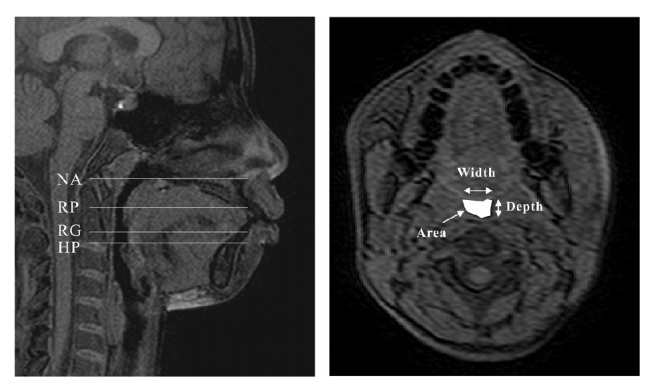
Landmarks and measurements of the upper airway on MRI. The nasopharynx (NA) was measured on the axial cross-section of the airway passing through the palatal plane. The retropalatal oropharynx (RP) was measured on the axial cross-section of the airway with the minimum area, between the palatal plane and the tip of the uvula. The retroglossal oropharynx (RG) was measured on the axial cross-section of the airway with the minimum area, between the tip of the uvula and the tip of epiglottis. The hypopharnx (HP) was measured on the axial cross-section of the airway passing through the tip of epiglottis.
